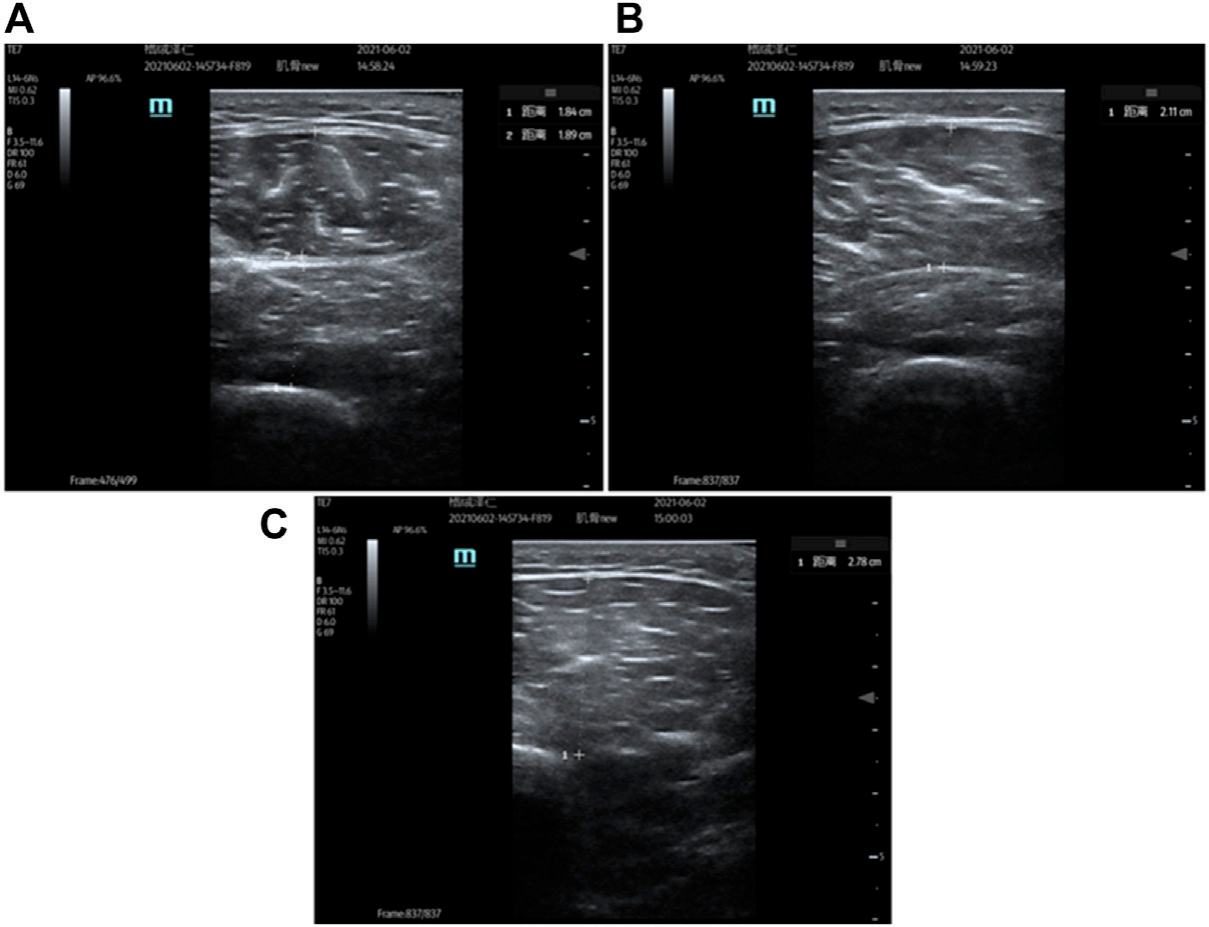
Ultrasound measurement of quadriceps thickness [(A): ultrasound measurement of the rectus femoris and vastus intermedius; (B) ultrasound measurement of the vastus lateralis; (C) ultrasound measurement of the vastus medialis].
